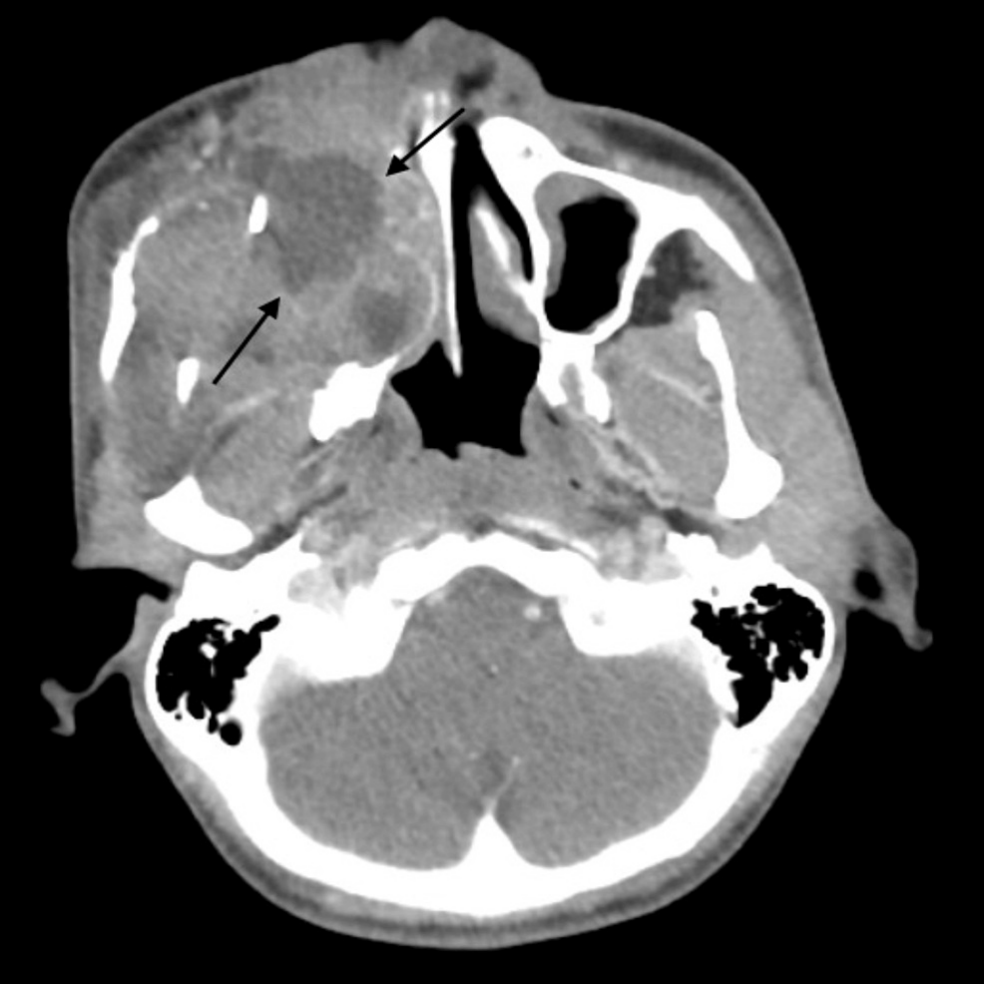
CT of paranasal sinuses taken in 2011 A large heterogeneously enhancing mass with solid and cystic components is seen in the area of the previously resected right maxillary sinus tumor measuring around 6.5 x 6.0 cm in its maximum dimensions. It erodes the adjacent bones. The lesion extends to the right nasal cavity and right ethmoidal air cells. Displaced surgical clips are seen in its anterior aspects The rest of the paranasal sinuses show partial mucosal thickening. Preservation of the fat planes between this lesion and the right orbital cavity is seen.
